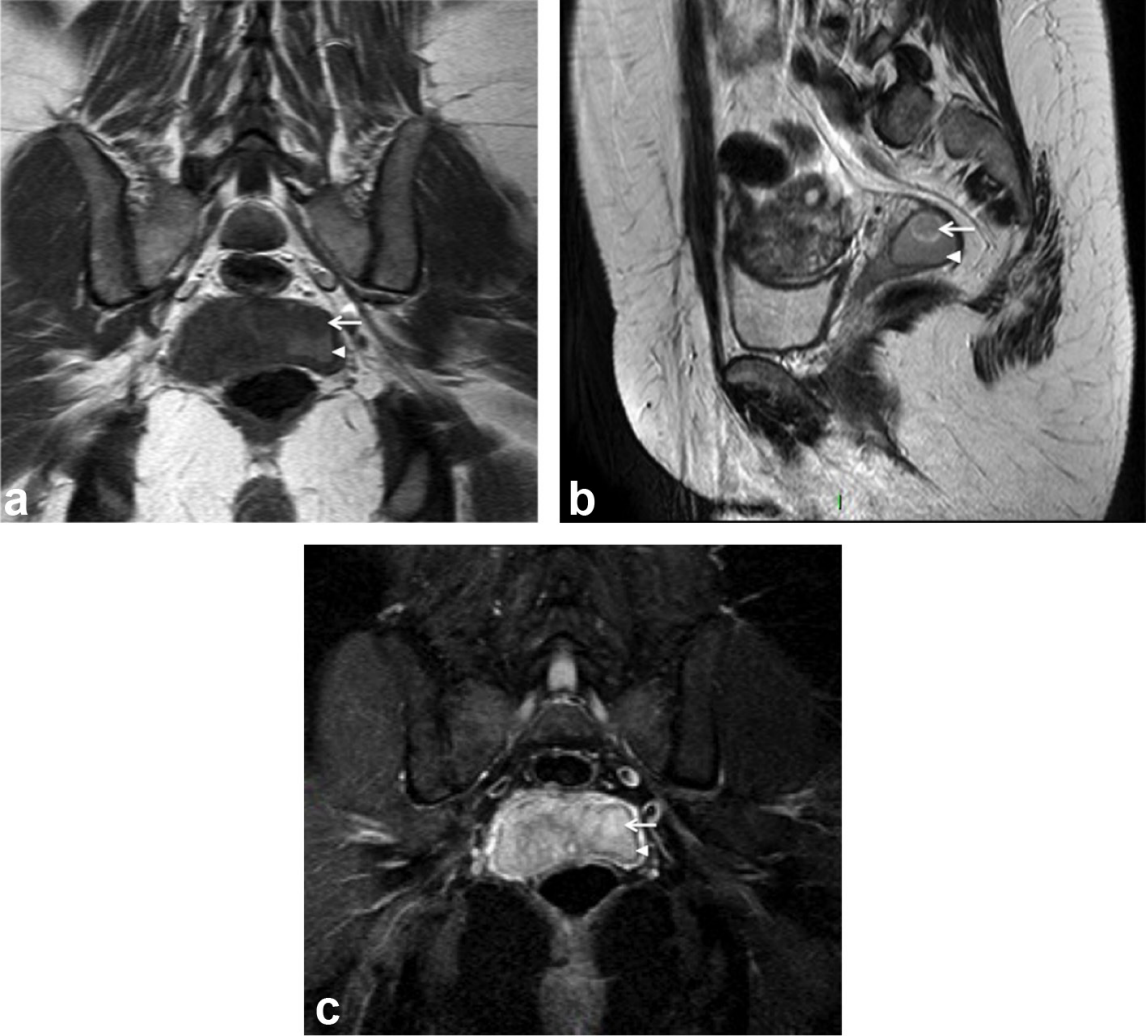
(a–c) Pelvic MRI study shows a well-defined cystic structure
